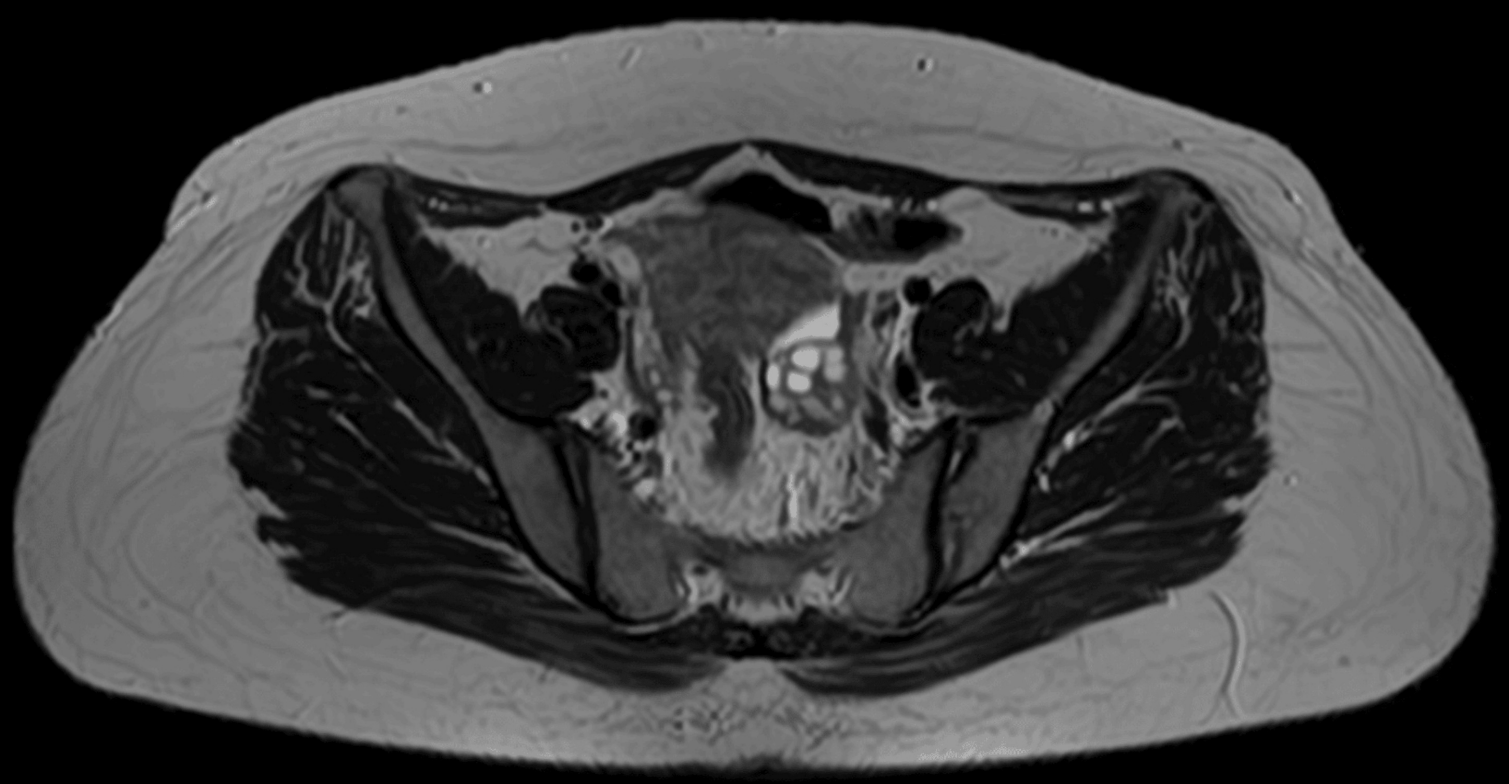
Axial magnetic resonance imaging of the pelvis six months after treatment, demonstrating a stable residual rectal lesion measuring 1.1 × 1.0 × 2.1 cm.
